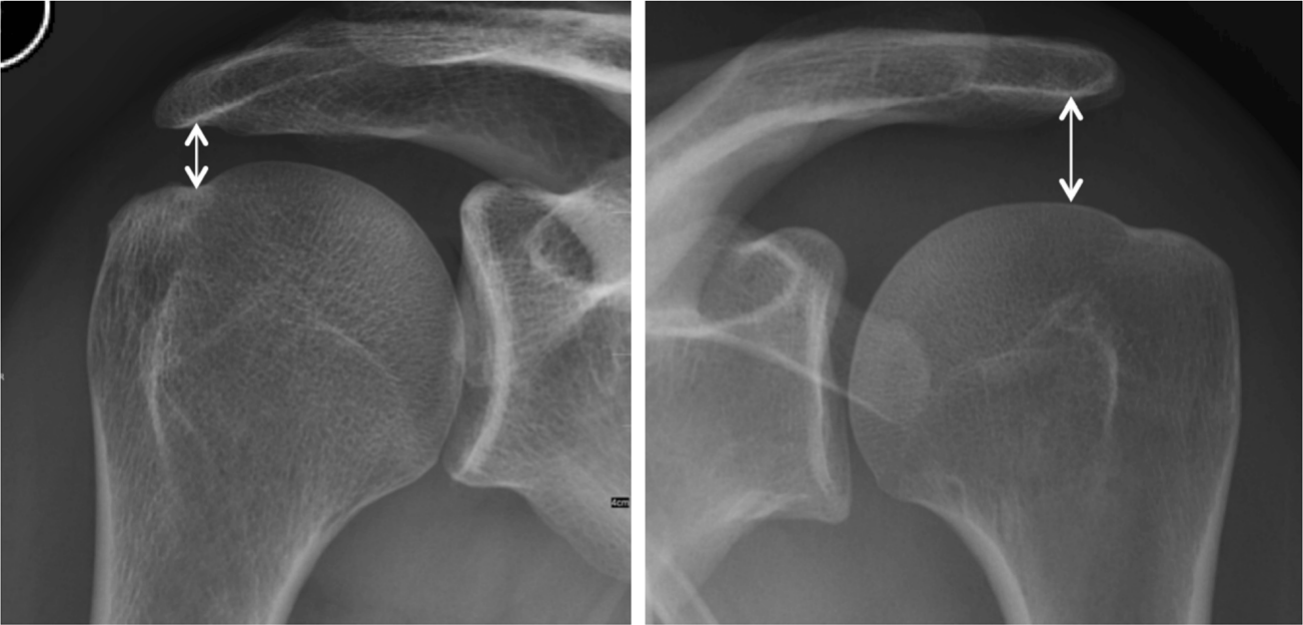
Anteroposterior radiographs of two shoulders in neutral rotation demonstrating that the acromiohumeral distance varies between individuals
